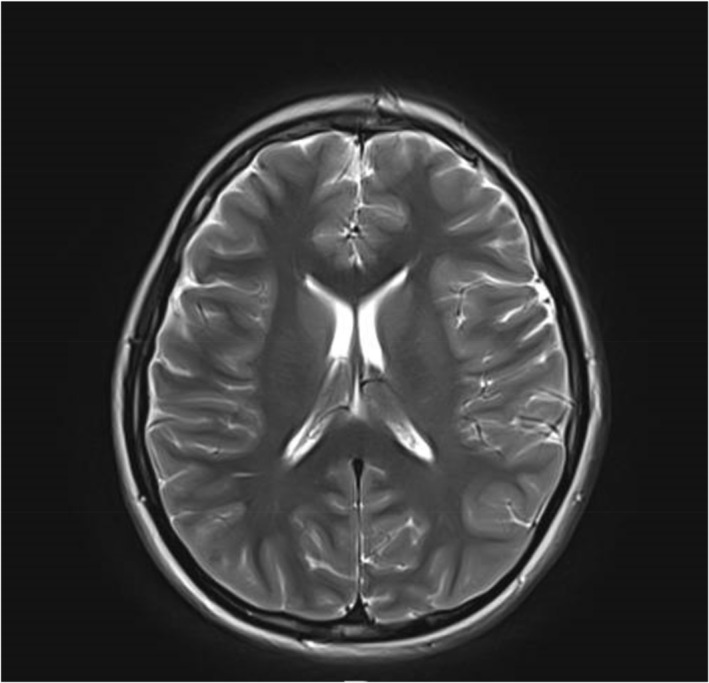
MRI brain did not reveal any significant abnormality. MRI, magnetic resonance imaging.
